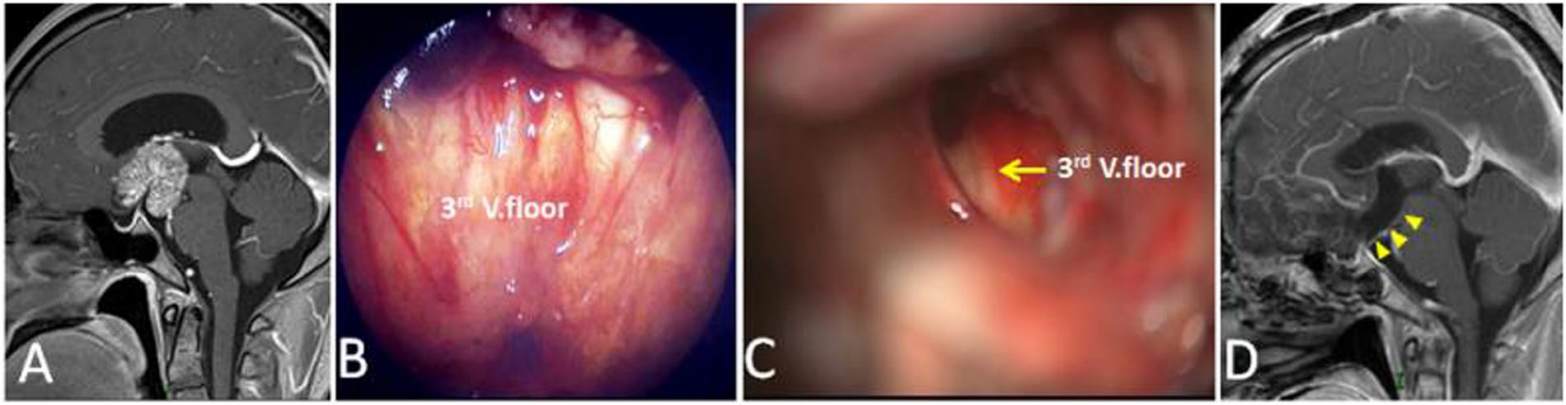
Example of a purely intraventricle Cp. (A) Sagittal postcontrast T1-weighted MR image showing a solid tumor only grows into the third ventricle. (B and C) Intraoperative photographs showing that the third ventricle floor was intact after tumor removal via the callosal-interforniceal approach. (D) Sagittal postcontrast T1-weighted MR images obtained after GTR. Yellow arrowheads indicate the intact the third ventricle floor. 3rd V., the third ventricle.
